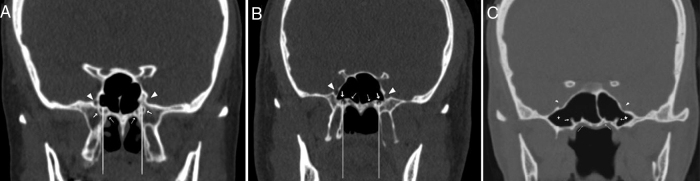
Coronal CT sections showing the vidian canal (thick arrow), palatovaginal canal (thin arrow), foramen rotundum (arrowhead), pterygoid recess pneumatization (star). (A) Bilateral vidian canals inside the sphenoid corpus (VC Type 1), the right vidian canal located lateral to medial pterygoid plate and left located at same line. (B) The vidian canals which partially protruding into the sphenoid sinus (VC Type 2) bilaterally located at same line with the medial pterygoid plate. (C) The vidian canals which totally inside the sphenoid sinus with a stalk (VC Type 3).
